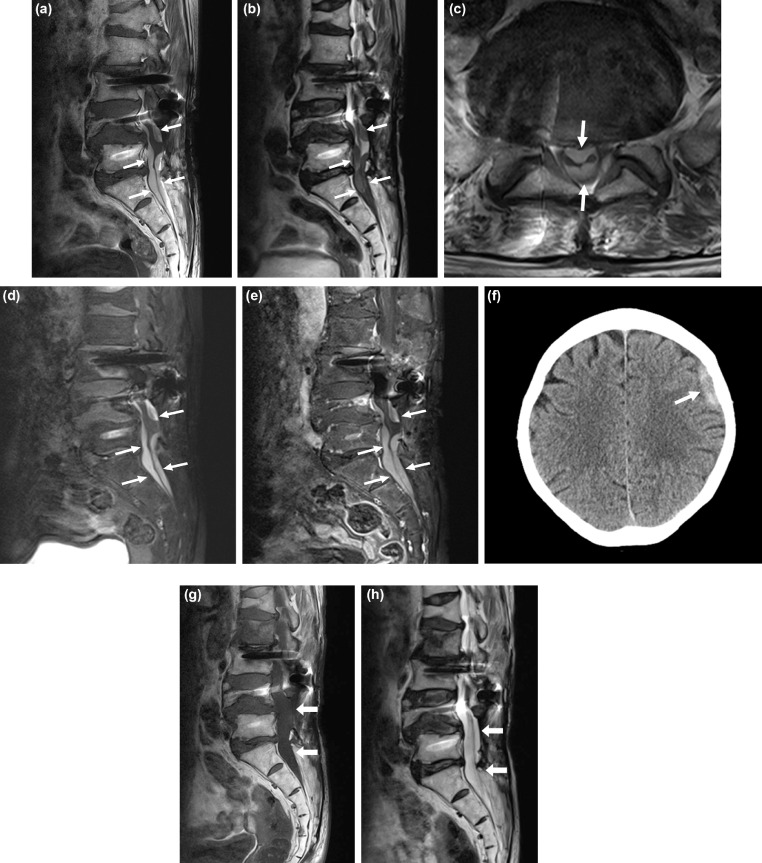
A 79-year-old woman with subacute spinal subdural hematoma and intracranial subdural hematoma. A and B. The sagittal T1-weighted (A) and T2-weighted (B) images show hyperintensity and hypointensity, respectively, of the subdural hematoma (arrows). Metal artifacts related to previous lumbar fusion and old compression fractures at the L1 and L4 are also seen. (C) The axial T1-weighted image confirming the subdural location of the hematoma (arrows). (D and E) Sagittal fat-saturated T1-weighted images without (D) and with (E) enhancement show hyperintensity of the hematoma (arrow). (F) Axial brain computed tomography (CT) image showing acute subdural hematoma on left convexity (arrow). (G and H) The sagittal T1-weighted (G) and T2-weighted (H) images show that the subdural hematoma had decreased in size remarkably after 10 months (arrows).
